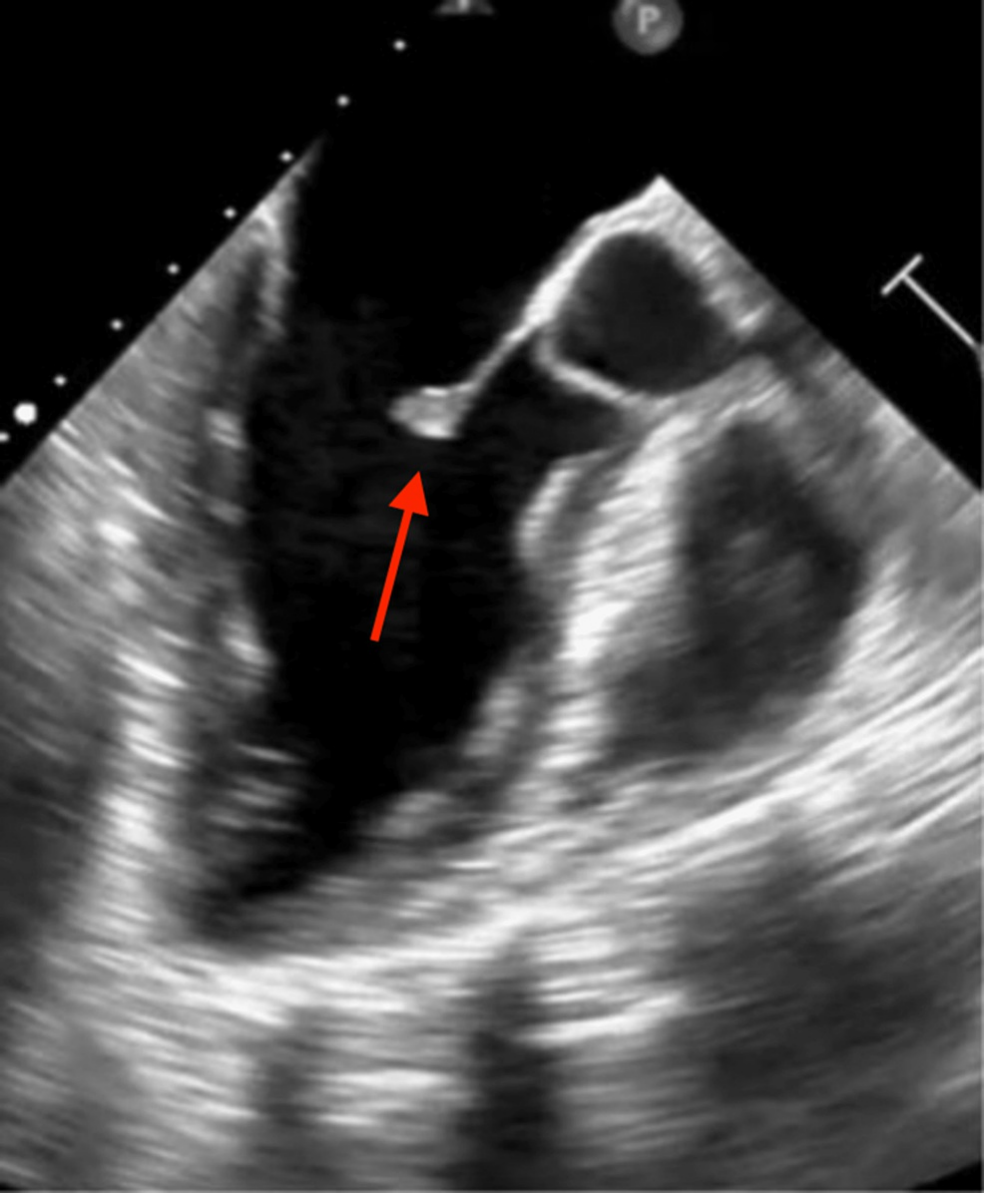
Three-month follow-up transesophageal echocardiogram demonstrating a more organized/nodular anterior mitral valve vegetation
